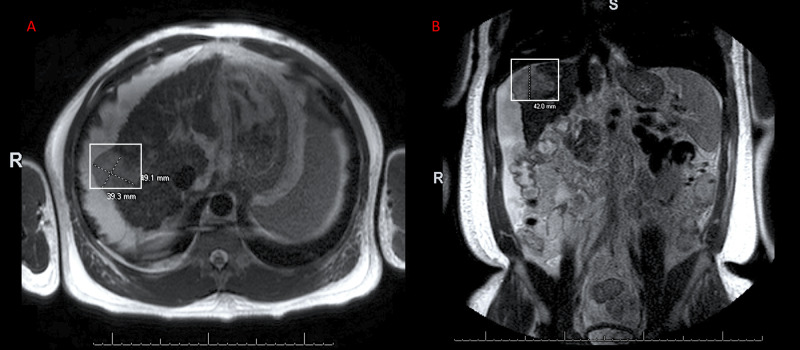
Exophytic Hepatic Mass Suggesting Hepatocellular Carcinoma Seen in MRI of the Abdomen with Contrast MRI (magnetic resonance imaging) of the abdomen with contrast demonstrates an exophytic mass, which measured 3.93 by 4.91 mm in the transverse view (Panel A) and 4.20 mm in the coronal view (Panel B).
